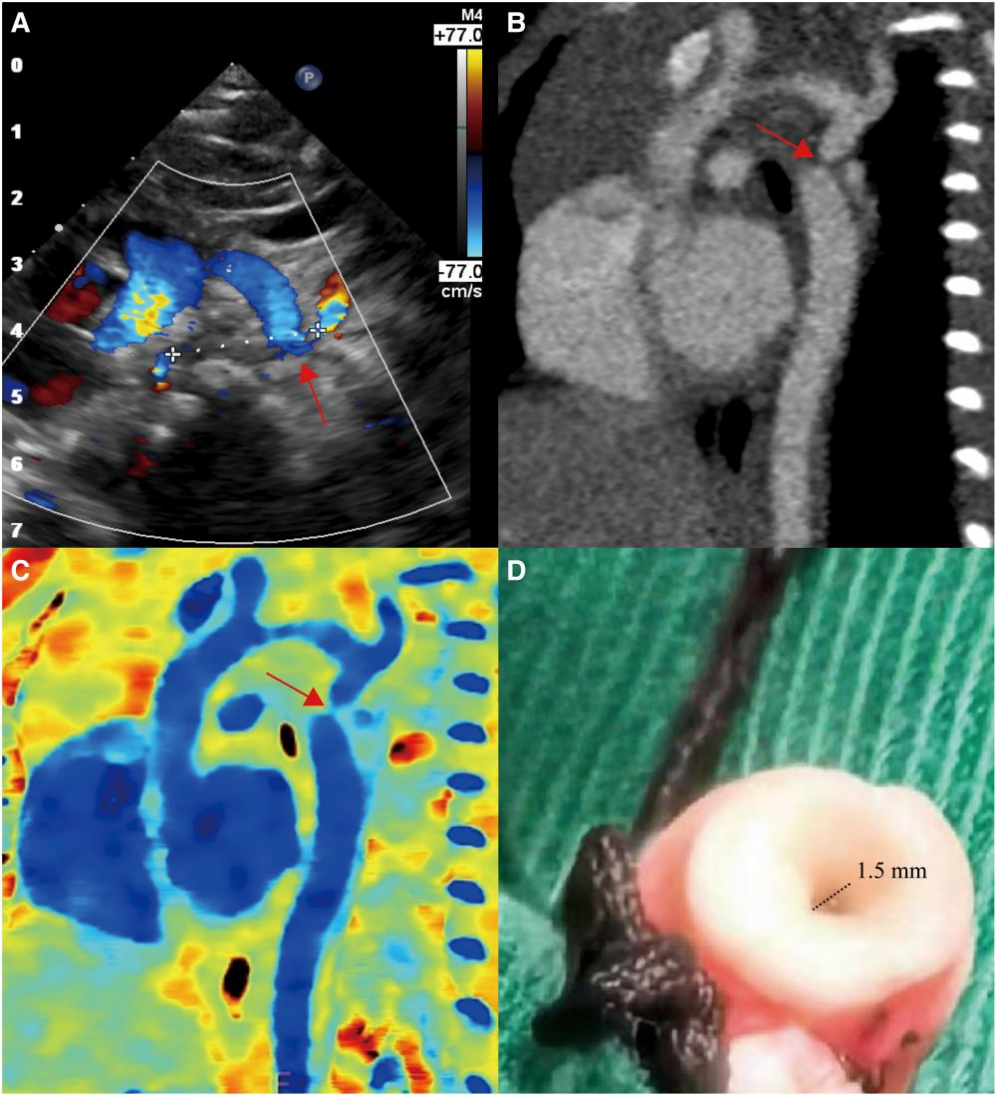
(*A*) Echocardiogram. The origin of the descending aorta, arrow. (*B*) Sagittal view of conventional CTA image. (*C*) Z-effective image. (*D*) Postoperative image of the resected stenotic segment with a diameter of 1.5 mm.
